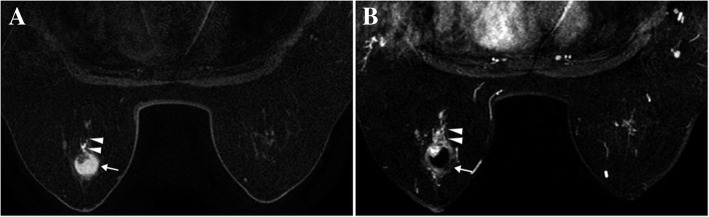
A case of papilloma with DCIS manifesting as a complex cystic mass (in 59-year-old woman). a The pre-contrast T1-weighted image reveals a mass (arrow) with nodular protrusion inside and duct dilation (arrowheads) in the left breast. b The MIP image of the subtracted images shows an enhanced complex cystic mass (arrow) and mixed mass-NME lesion (arrowheads). The pathology is intraductal papilloma with DCIS
